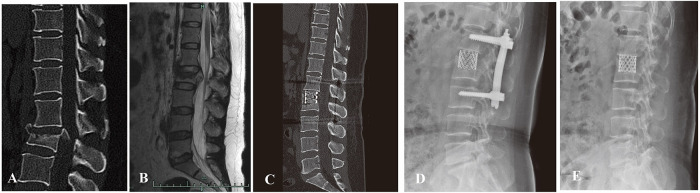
(A,B) preoperative sagittal CT scan and MRI images of a 42-year-old male patient with L2 burst fracture with nerve deficit. (C) Postoperative lateral CT image demonstrating good correction of kyphotic deformity *via* posterior instrumentation combined with AIPA anterior decompression and titanium cage placement. (D) An x-ray image was taken 3 months after surgery. (E) x-ray image demonstrating that the posterior screw–rod system had been removed.
